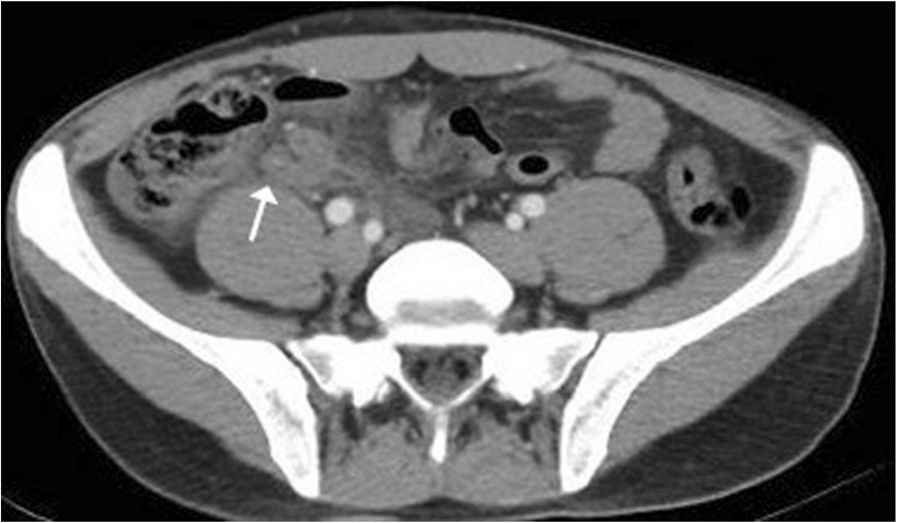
Abdominal CT scan. In the right lower quadrant, remnant appendix tissue in the pericecal region and fecalitis within the appendix tissue are observed
